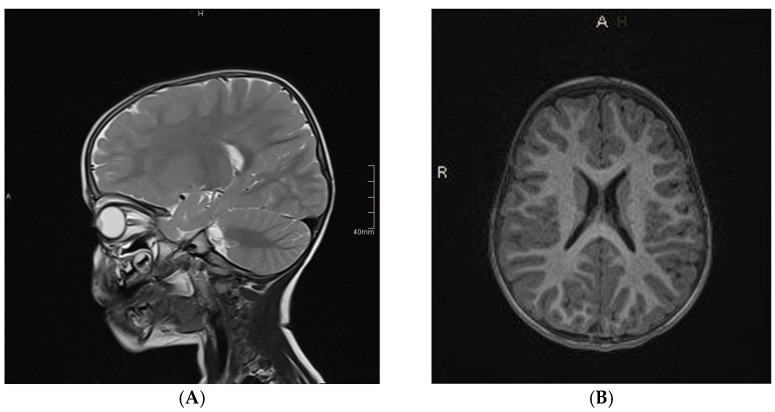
Head MR imaging (T2−weighted (A) and T1−weighted (B) images) of 2.5−year−old boy with a lack of eye contact and abnormal eye movements. The MR examination was normal. Source: own study.
